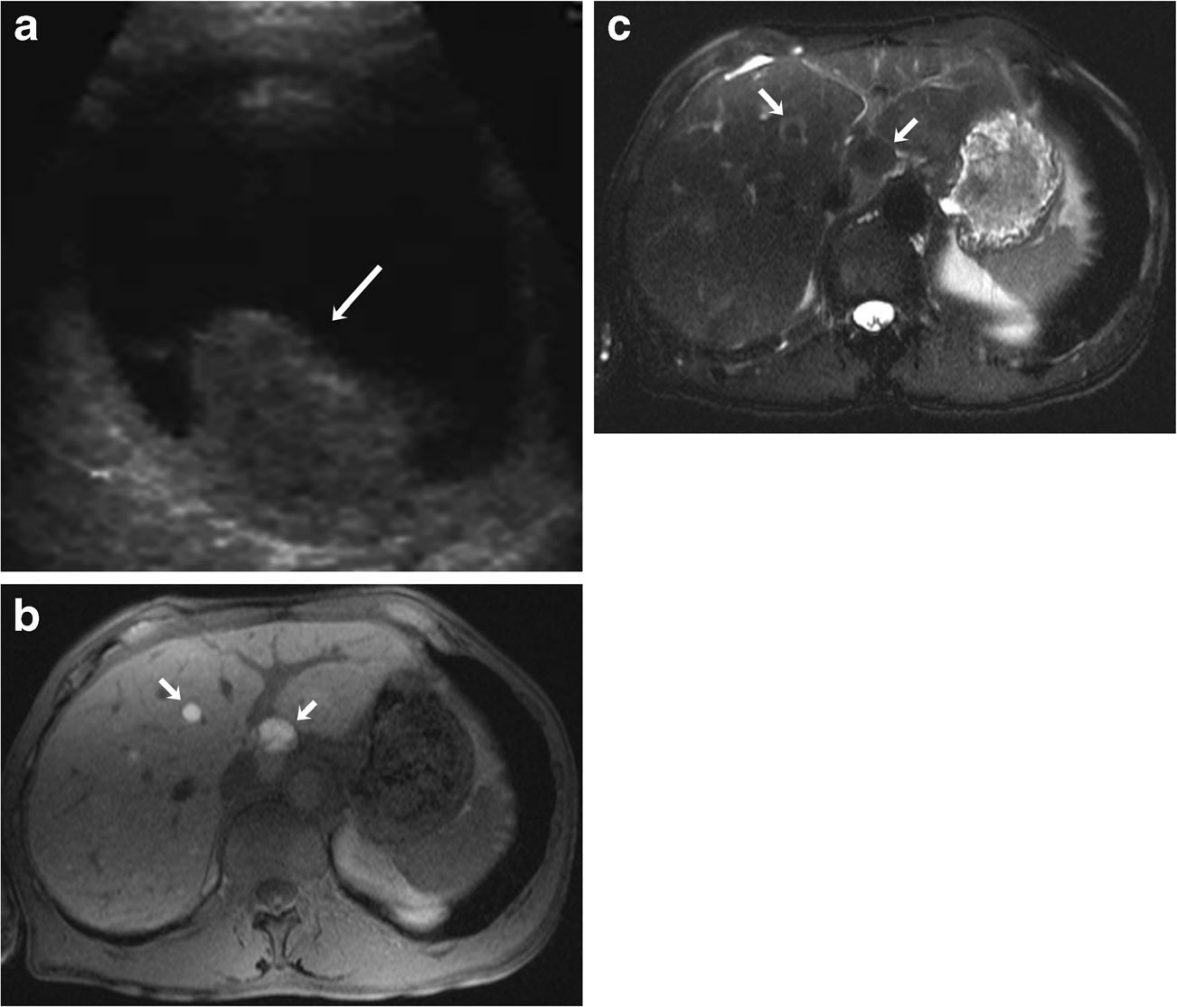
A 52-year-old female with uveal melanoma and hepatic metastases. a Grey-scale ultrasound image showing an echogenic mass in the posterior segment of the left globe (arrow). b Axial fat-suppressed T1-weighted MR image showing hyperintense hepatic metastatic lesions (arrow). c The lesions are hypointense on the corresponding axial fat-suppressed T2-weighted MR image (arrows)
